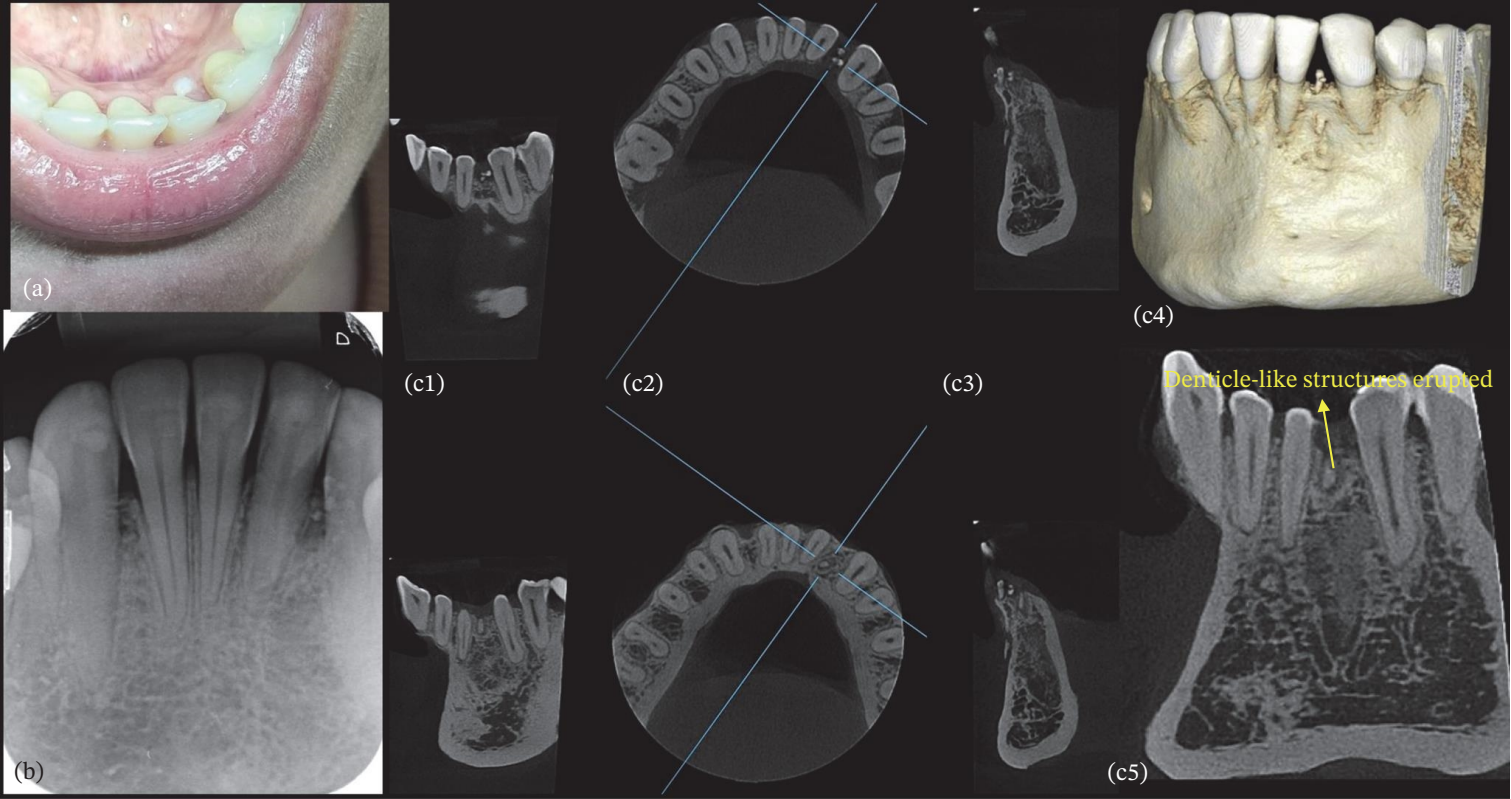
Clinical and tomographic follow‐up. (a) Eruption of the supernumerary tooth in the lingual region. (b) Postoperative radiographic follow‐up showing residual denticle‐like structures. (c) Coronal (c1, c5), axial (c2), and sagittal (c3) planes, as well as three‐dimensional reconstruction (c4), CBCT follow‐up, demonstrating the presence of residual denticle‐like structures and their proximity with adjacent permanent teeth.
